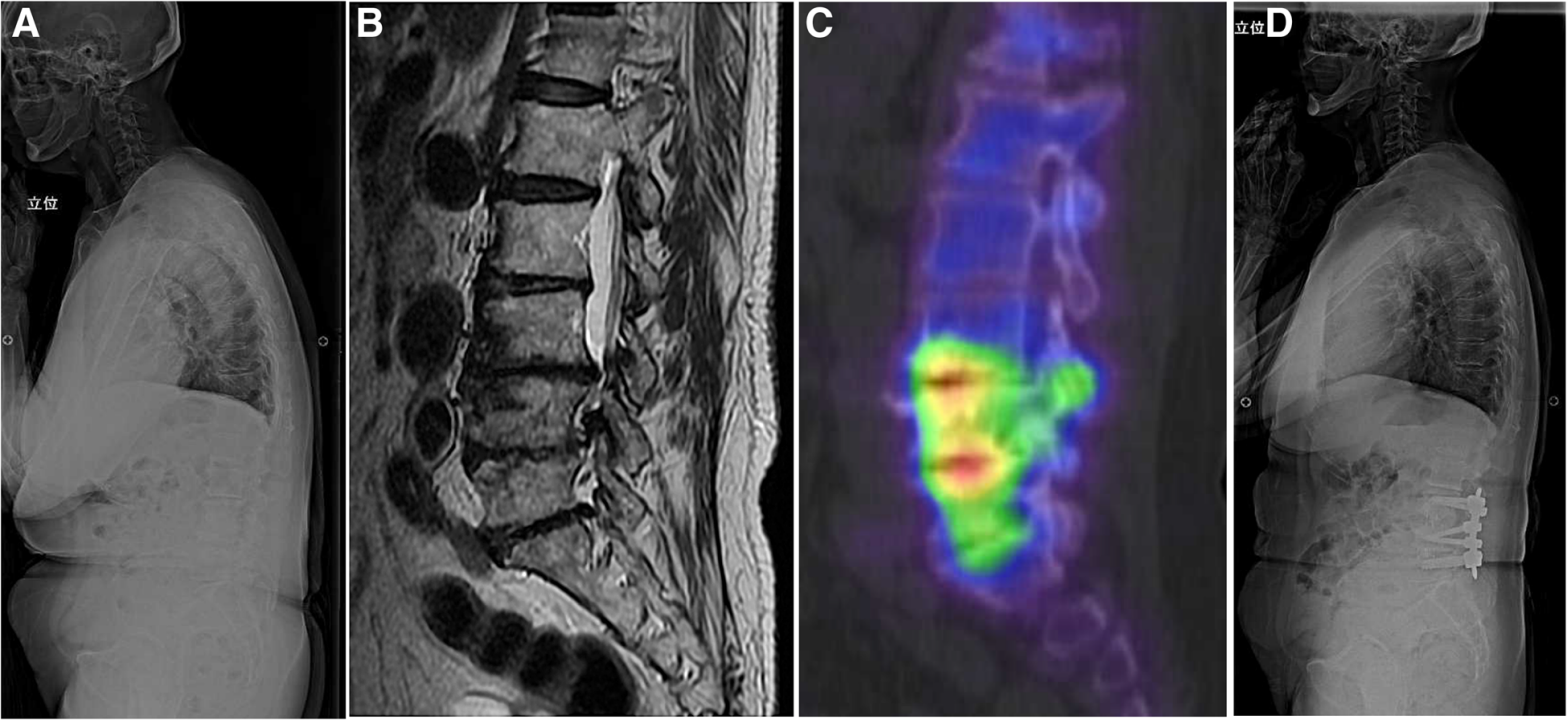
A 68-year-old woman with degenerative lumbar discopathy. a Lateral view of radiograph showing adult spinal deformity with a sagittal imbalance. b T2-weighted MR sagittal image of the lumbar spine without remarkable findings indicating the site of pain-causing pathology. c Bone SPECT/CT image of the lumbar spine showing a hotspot in the vertebral disks of L3/4 and L4/5. d Lateral view radiograph after oblique lumbar interbody fusion and percutaneous pedicle screw fixation. MR, magnetic resonance; SPECT/CT, bone scintigraphy with single photon emission computed tomography
